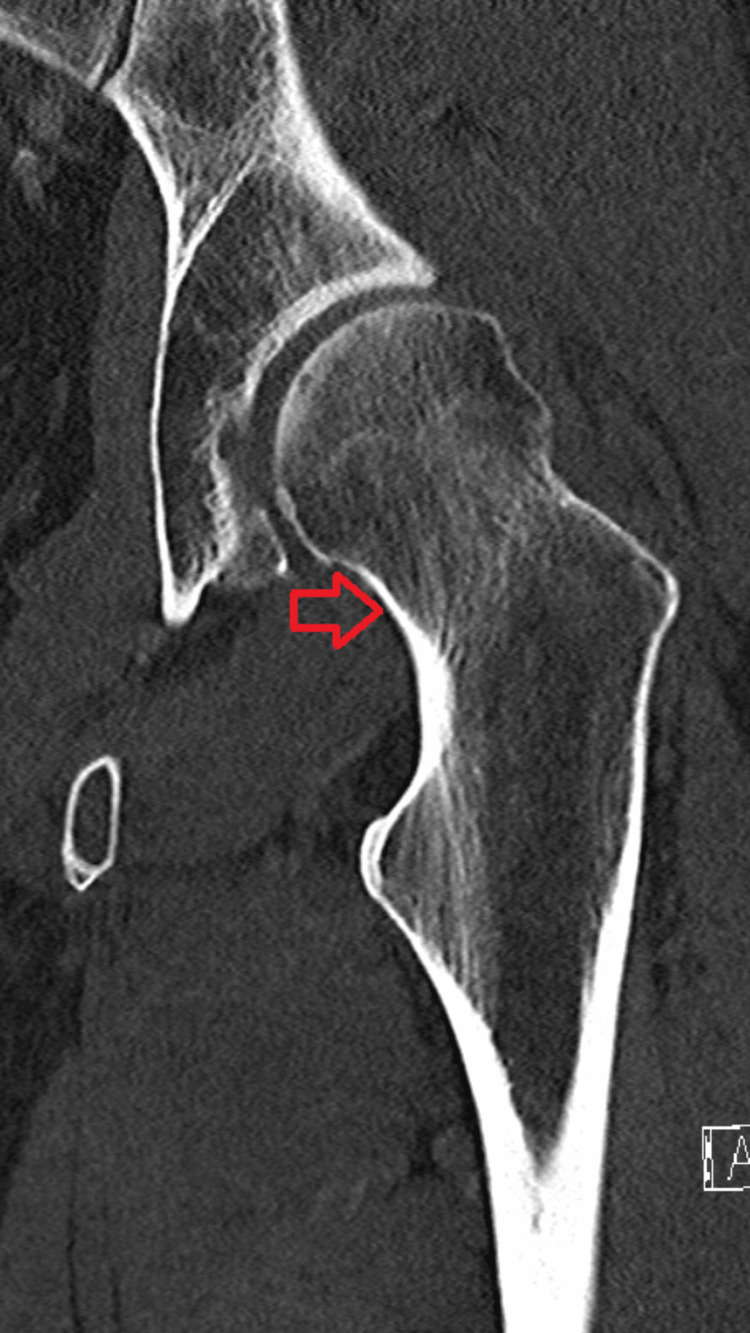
CT image of the left hip, coronal view The CT reveals partial uncovering of the left hip with evidence of dysplasia and enlargement of the femoral neck (red arrow).
